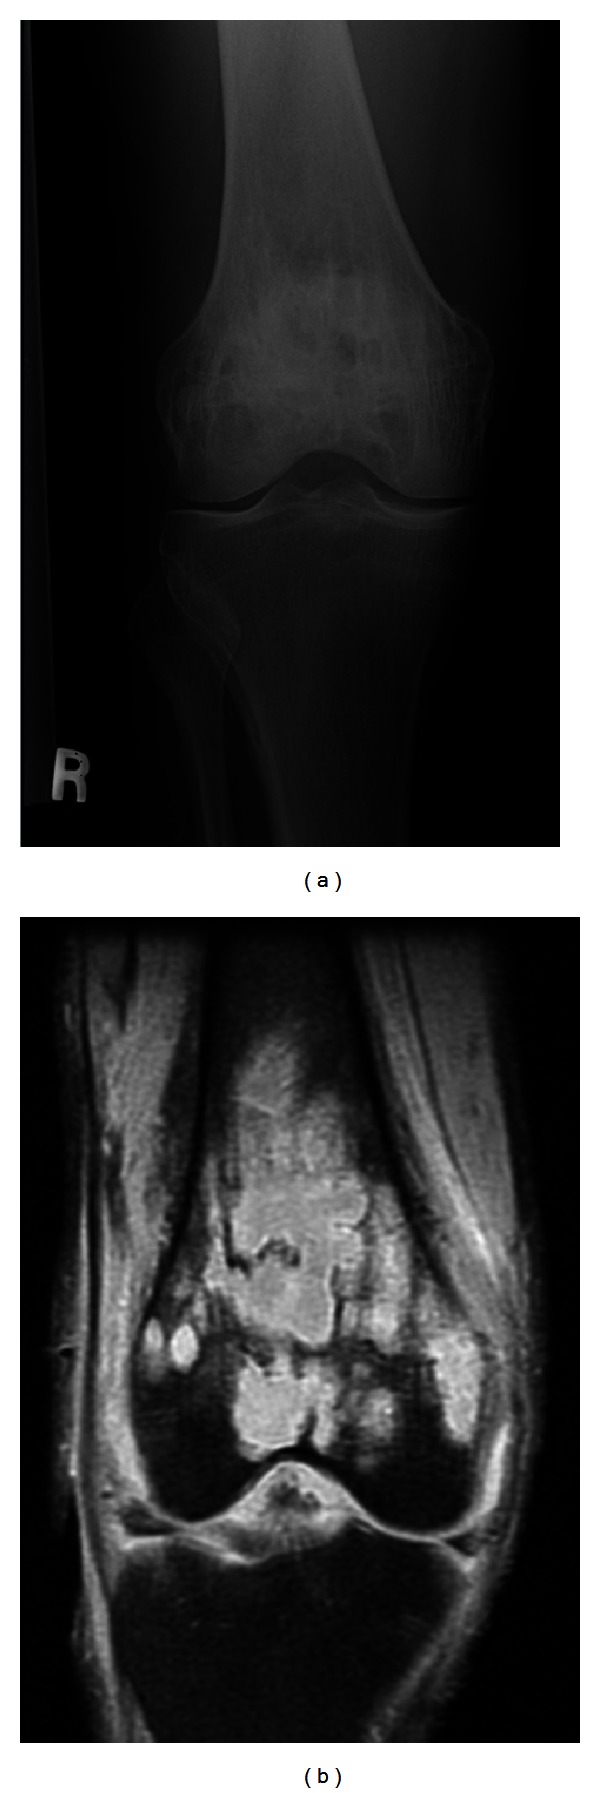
(a) and (b) showing X-ray and MRI, respectively.
